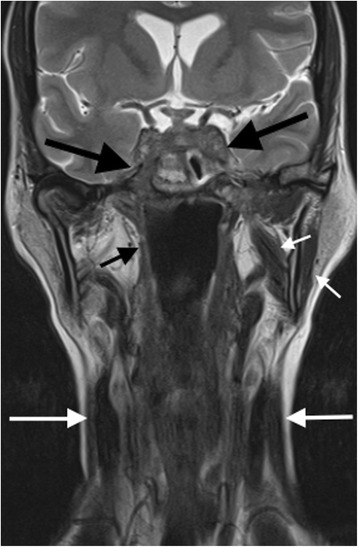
Coronal T2-weighted MRI image of the skull base and neck (pre-cyclophosphamide treatment). There is severe chronic inflammatory soft tissue abnormality affecting the cavernous sinuses and central and lateral skull base, including the left cavernous sinus and right sphenoid bone and foramen rotundum (large black arrows). Secondary cranial nerve palsies are present. Palsy of the mandibular division of the right trigeminal nerve has resulted in atrophy of the right masticator space muscle, in comparison to the more normal muscle volume on the left (short white arrows). Lower cranial nerve palsies are demonstrated by atrophy of the right superior pharyngeal constrictor muscle (short black arrow) and sternocleidomastoid muscles (long white arrows)
